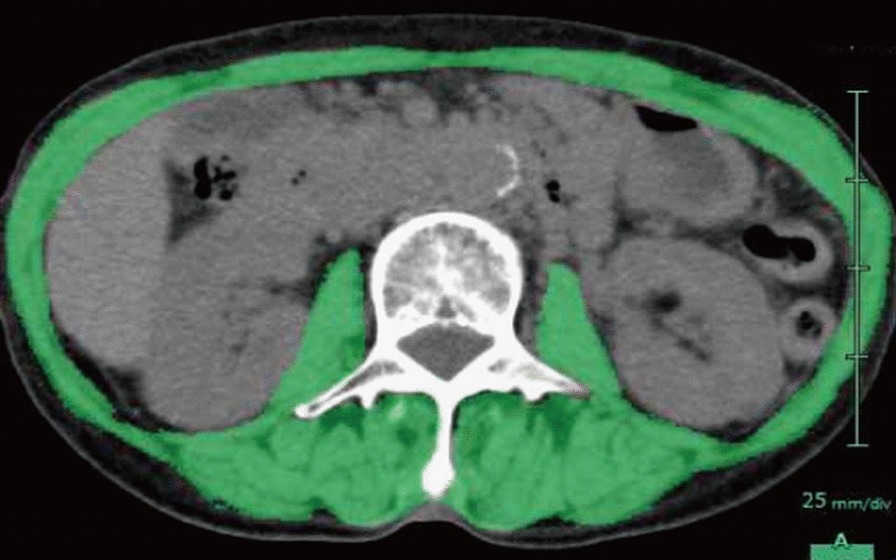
Representative of CT scan image. After skeletal muscle area was estimated by subtracting the areas of visceral fat and subcutaneous fat from the total area, it was determined on CT by manual tracing (green area). The areas of visceral and subcutaneous fat were calculated by the abdominal application software, automatically
